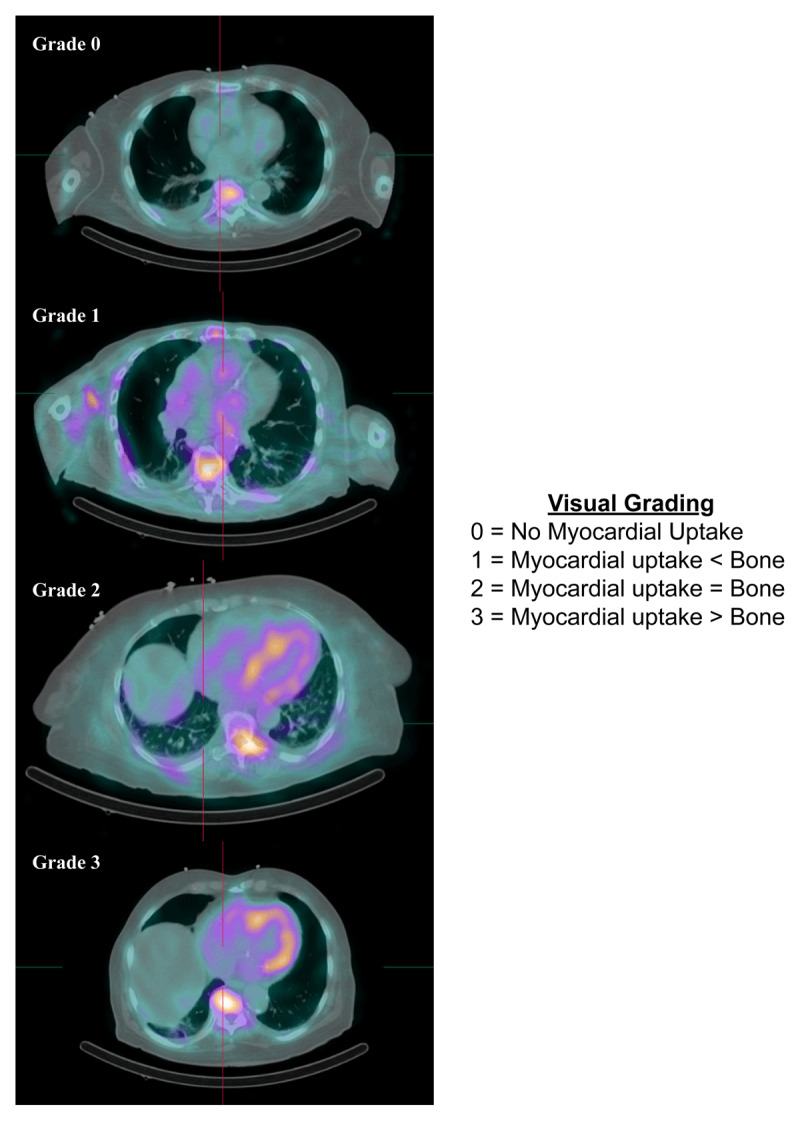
^99m^Tc-PYP cardiac scintigraphy using single photo emission computer tomography/computed tomography visual scoring. Reproduced with permission from Springer Nature. doi: *10.1007/s10741-021-10174-x*
